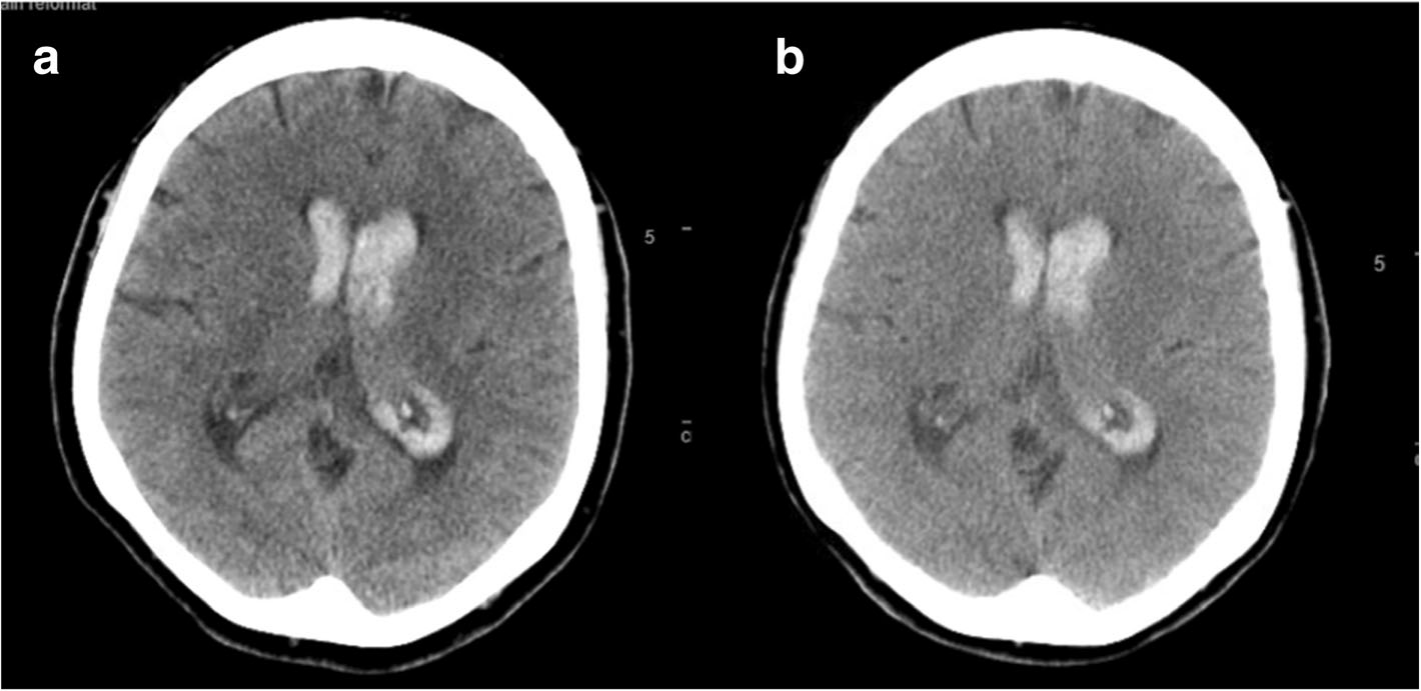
Noncontrast head CT demonstrated intraventricular hemorrhage (IVH) a Initial head CT showing diffuse IVH in the bilateral lateral ventricles. b Follow-up head CT showing stable IVH
